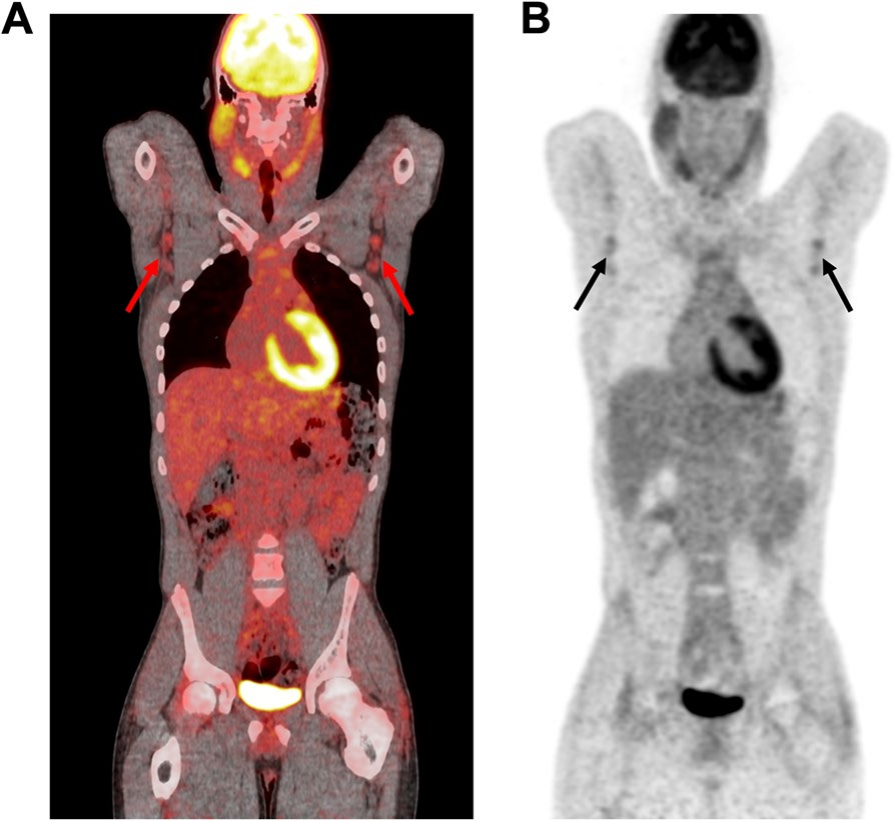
Whole body 18F-FDG PET/ ultra low dose CT in a 11 year-old patient revealed abnormal 18F-FDG uptake not specific for an active granulomatous disease. A left axillary lymph node was biopsied and definite ocular sarcoidosis was diagnosed. A FDG PET/CT Fusion Coronal View. B FDG PET coronal view. Red and black arrows: Low hypermetabolic axillary bilateral lymph nodes
